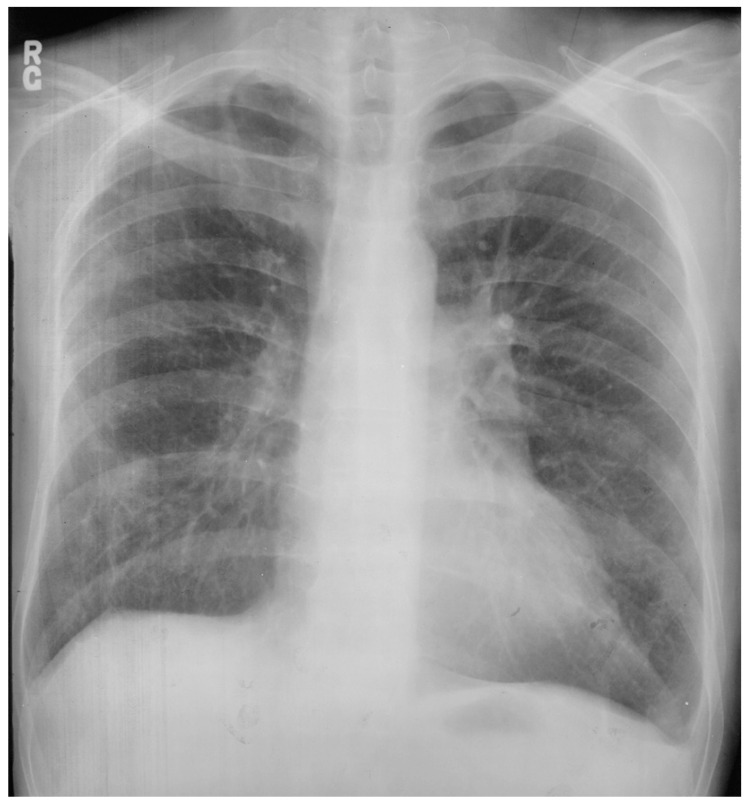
Posteroanterior chest X-ray of congenital absence of the right pulmonary artery: This is the chest X-ray of a 43-year-old male who sought another opinion because, after a sudden illness, he was dependent on supplemental oxygen. He was a coal miner who collapsed in the mine with chest pain and dyspnea one month before this CXR was done. When acutely ill he was taken to the hospital and found to have multiple pulmonary emboli to the lower left lung and no perfusion of the right lung. He was treated with intravenous heparin and warfarin. His chest pain resolved but he was not able to wean off the supplemental oxygen due to dyspnea at rest. This posteroanterior chest X-ray is well centered and there is a good inspiration. The cardiothoracic ratio is normal. The entire right lung is hypolucent with decreased vascularity in the left lower lung, compared to the left upper lung. There is pleural thickening at the left costophrenic angle. These left lung and left pleural findings are probably residua of the pulmonary emboli. Physical examination was not revealing. He underwent digital subtraction angiography with contrast injection into the right atrium. It showed congenital agenesis of the right pulmonary artery. The further compromise of congenitally halved pulmonary artery circulation by the pulmonary emboli rendered this man hypoxic and dyspneic. He was encouraged that due to the good medical care he received, he survived a life-threatening illness. Now that he requires supplemental oxygen he can no longer work in the coal mine.
